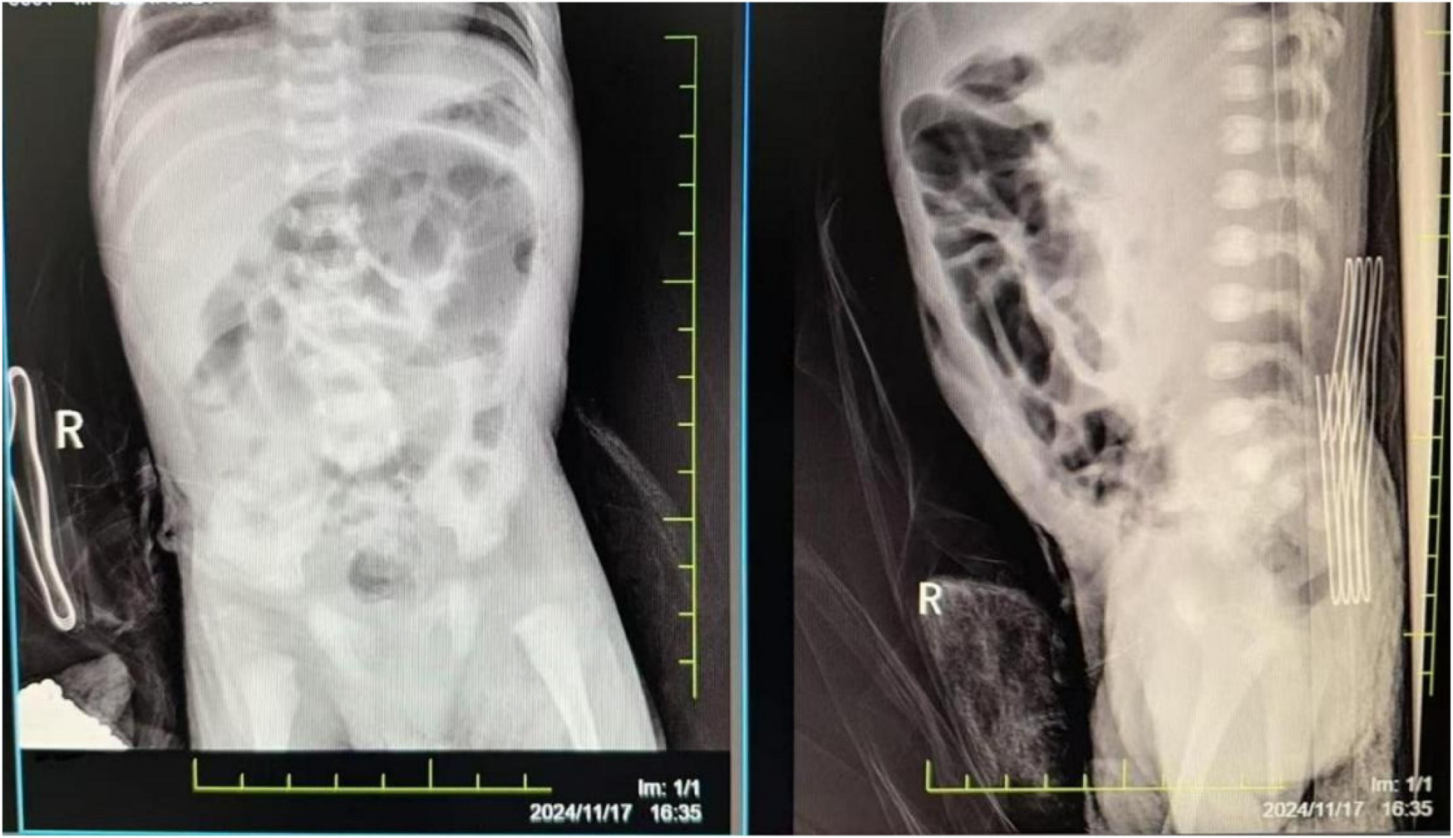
The abdominal radiograph on postoperative day 21 suggests recurrent necrotizing enterocolitis.
